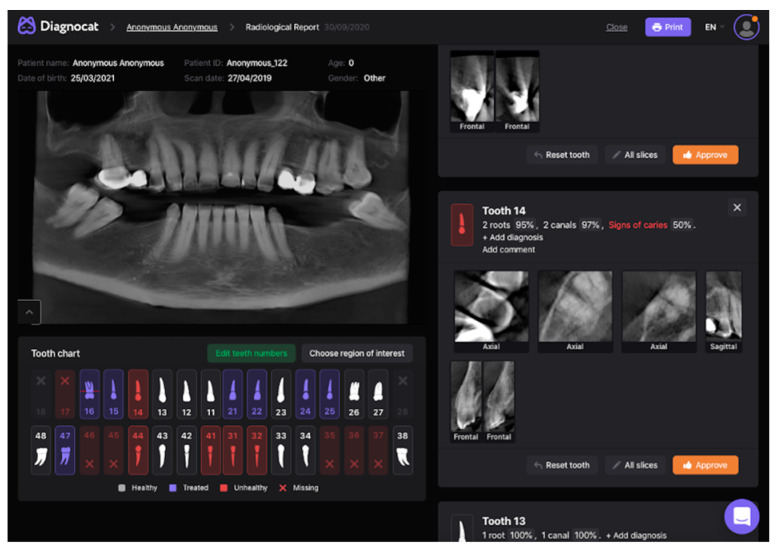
Interface for aided evaluation (Diagnocat). On the left side, at the top, the synthetic–panoramic image produced from the CBCT volume is provided for an overall view, while underneath, there is a dental chart that provides information about the condition of each tooth. The colors of white and purple represent a healthy and treated tooth, while the red means an unhealthy or missing tooth. On the right, the predictions of the system for the relevant tooth are provided by image slices in different axes.
